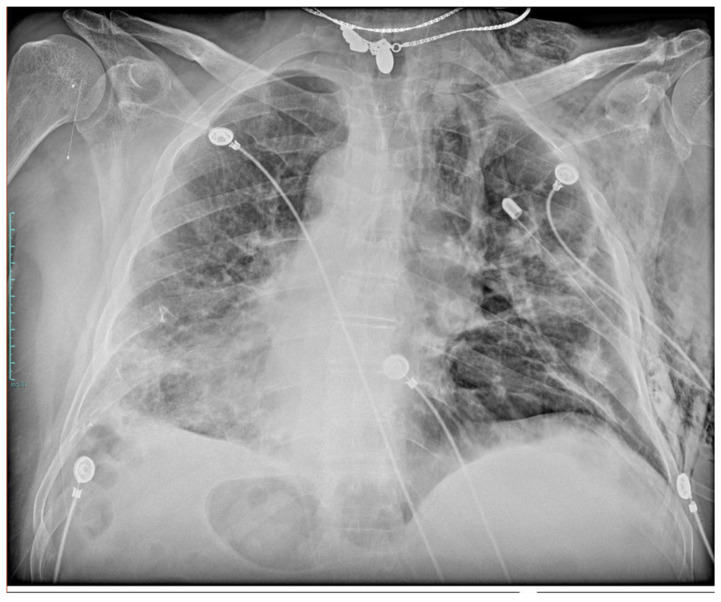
Chest X-ray showing right-sided chest tube placed for pneumothorax and mediastinal emphysema in COVID-19 patient.
